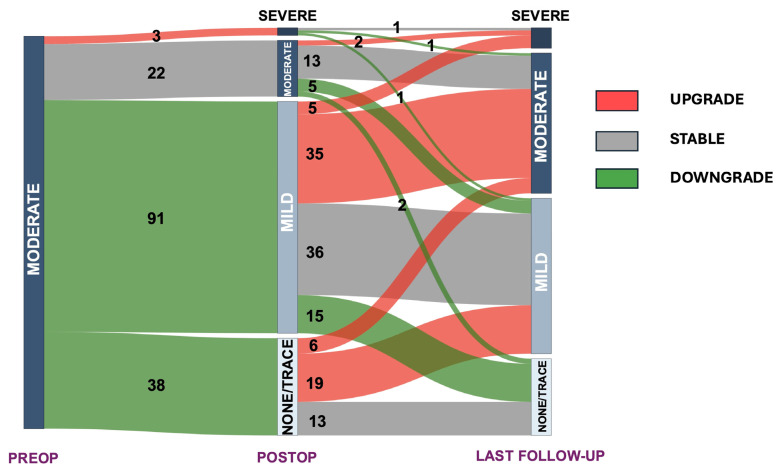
Sankey plot of mitral regurgitation progression from preoperative (moderate) to last echocardiographic follow-up. Numbers represent number of patients and mitral regurgitation evolution is color-coded: red = upgrade; gray = stable disease; green = downgrade.
